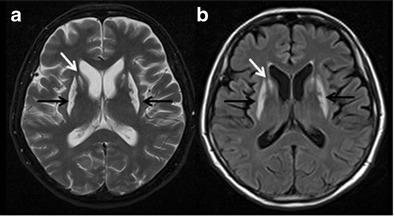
Methanol toxicity. A 52-year-old female with a history of accidental methanol ingestion, presented with cognitive decline and rigidity in the extremities. a & b axial T2W and FLAIR images of the brain show necrosis of bilateral putamen (*black arrows*) and head of caudate nuclei (*white arrow*)
